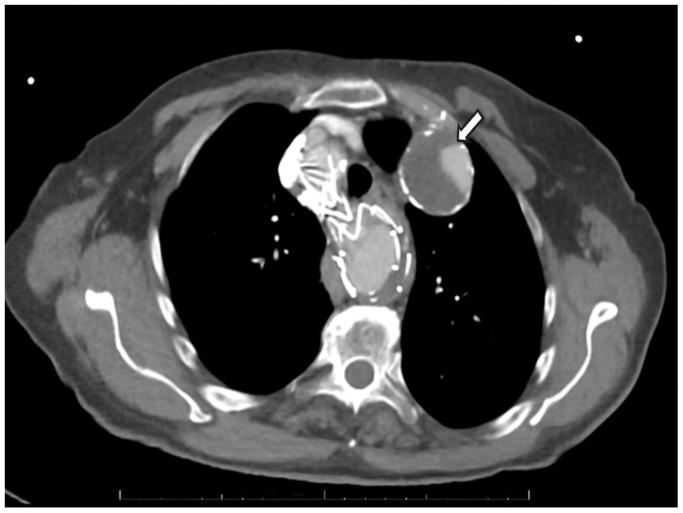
Status post 1 month computed tomography angiography (CTA) imaging in the axial plane demonstrating persistent type II endoleak (*white arrow*) before additional interventions.
